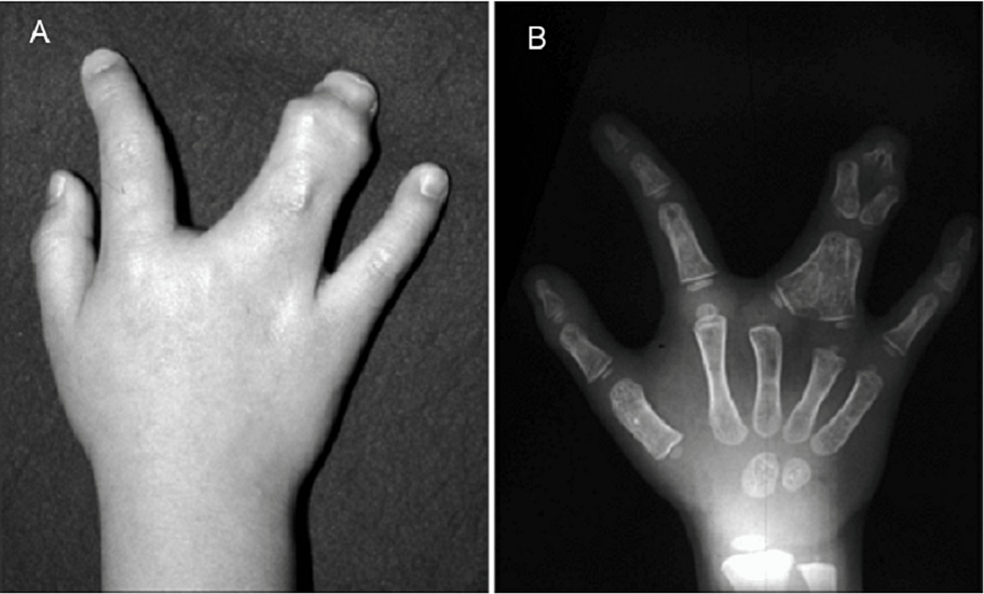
(A) Right-hand syndactyly between the distal and proximal, but not the intermediate, phalanges; (B) Radiograph of right-hand syndactyly Open access images courtesy of Ogino et al. [9]
